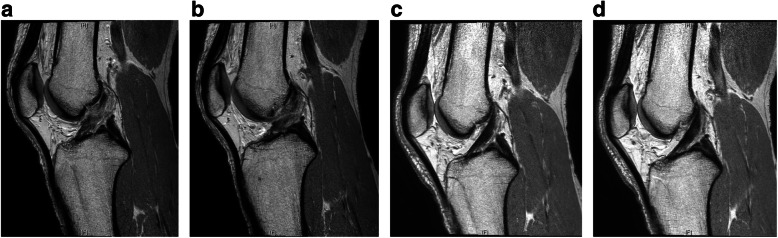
Non-operative treatment was performed on the 28 year-old male patient with an acute anterior collateral ligament (ACL) injury that had occurred while playing soccer 1 week ago. a, b T2-weighted magnetic resonance (MR) sagittal image of the knee indicates a midsubstance ACL rupture. Initial physical examination was Lachman test grade 1 and pivot-shift test grade 1, and the Tegner activity score before the injury was 7.0. c, d T2-weighted MR image of the same patient treated non-operatively according to our program shows a well-healed ACL at 1 year after injury. At 2 years after the injury, physical examination was Lachman test grade 0 and pivot-shift test grade 0, and the Tegner activity score was 7.0
